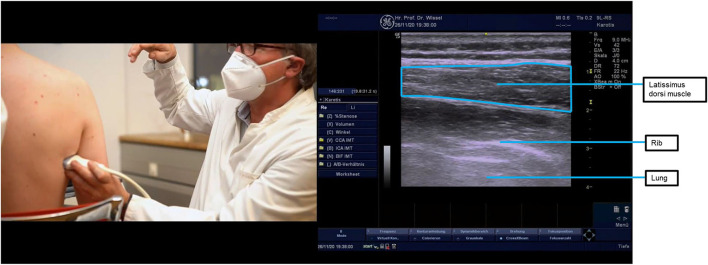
Ultrasound probe placement and image of the latissimus dorsi muscle. The latissimus dorsi muscle can be located by beginning at the posterior axillary fold and then moving the ultrasound probe down the thorax, about halfway toward the lower thoracic vertebrae. To better distinguish the muscle from the subcutaneous tissue, the probe should be parallel to muscle fiber orientation, thus making its fibrillar pattern apparent. In this anatomical location, the latissimus dorsi will be the most superficial muscle, as shown in the image. Underneath it lies the serratus anterior muscle, also shown in the ultrasound image. Just under the serratus anterior muscle, it is also possible to see one rounder hyperechoic line on the bottom right, which corresponds to a rib, and a more linear hyperechoic line on the bottom left, which corresponds to the pleura. The injection can be performed with the probe either in the longitudinal or transversal orientations, but longitudinal is preferred due to the proximity of the pleura. Multiple injections (two or three) are recommended for the latissimus dorsi due to the size and strength of the muscle.
